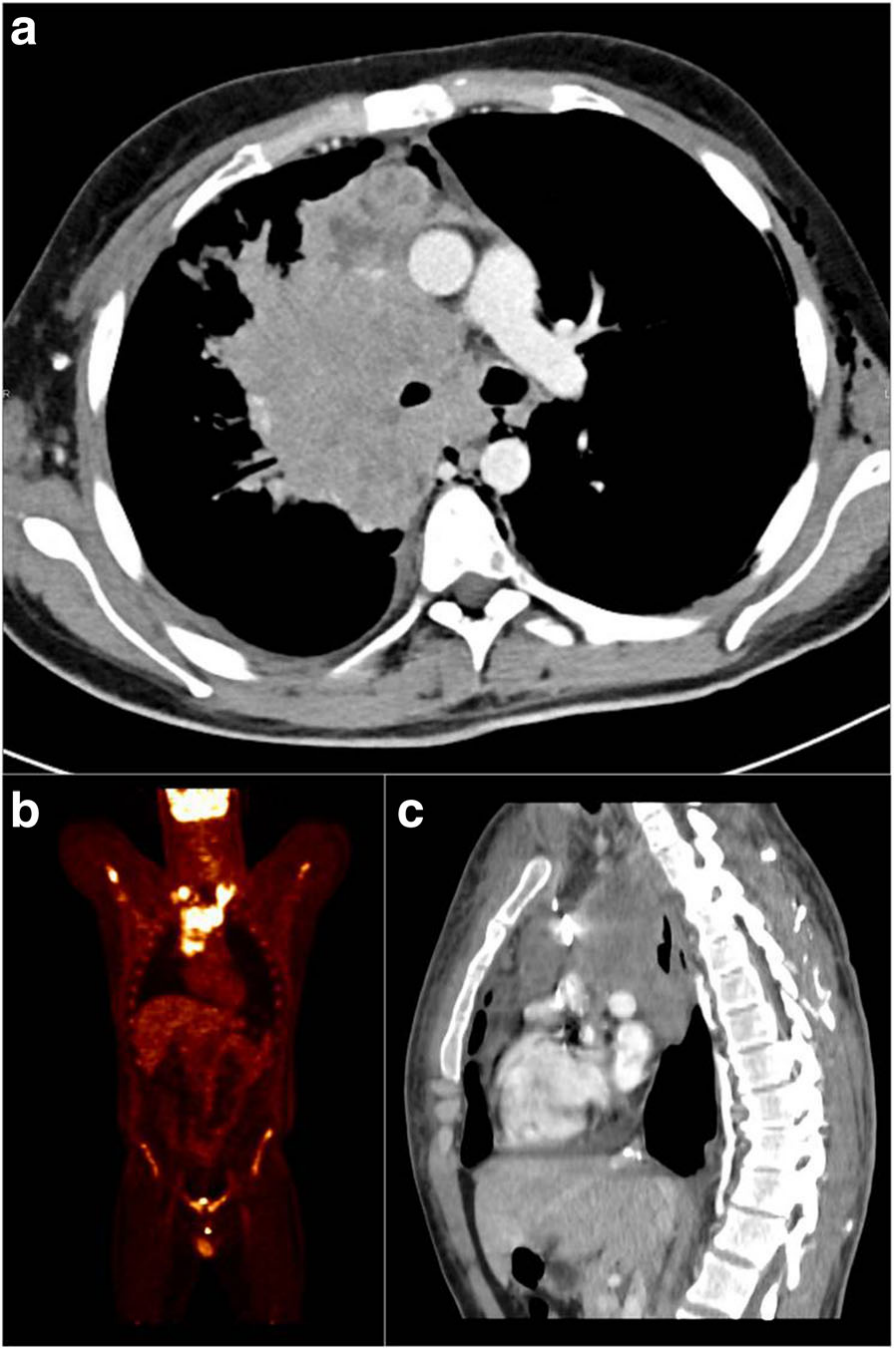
Disease status at diagnosis. Panel a, c: CT scan showing bulky mediastinal mass with involvemente of right lung ilum, compression of the right superior bronchus, and dislocation of the trachea and right intermediate bronchus with pleural and pericardial effusion (panel a axial, panel c sagittal). Panel b: PET scan showing supraclavicular involvement of the disease and absence of liver metastases. CT, computed tomography; PET positron emission tomography
